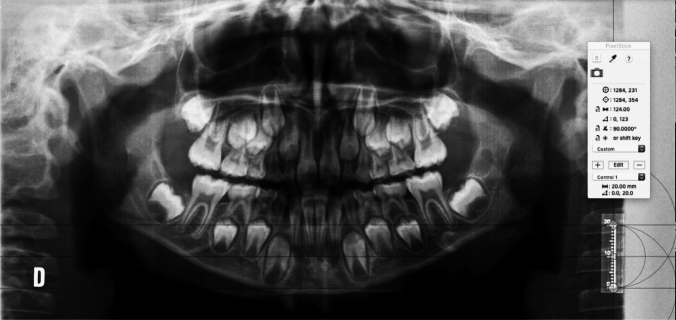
Checking the X-ray calibration. When the cursor is placed during the calibration, these parameters must match before moving on to the next step (20 mm)
